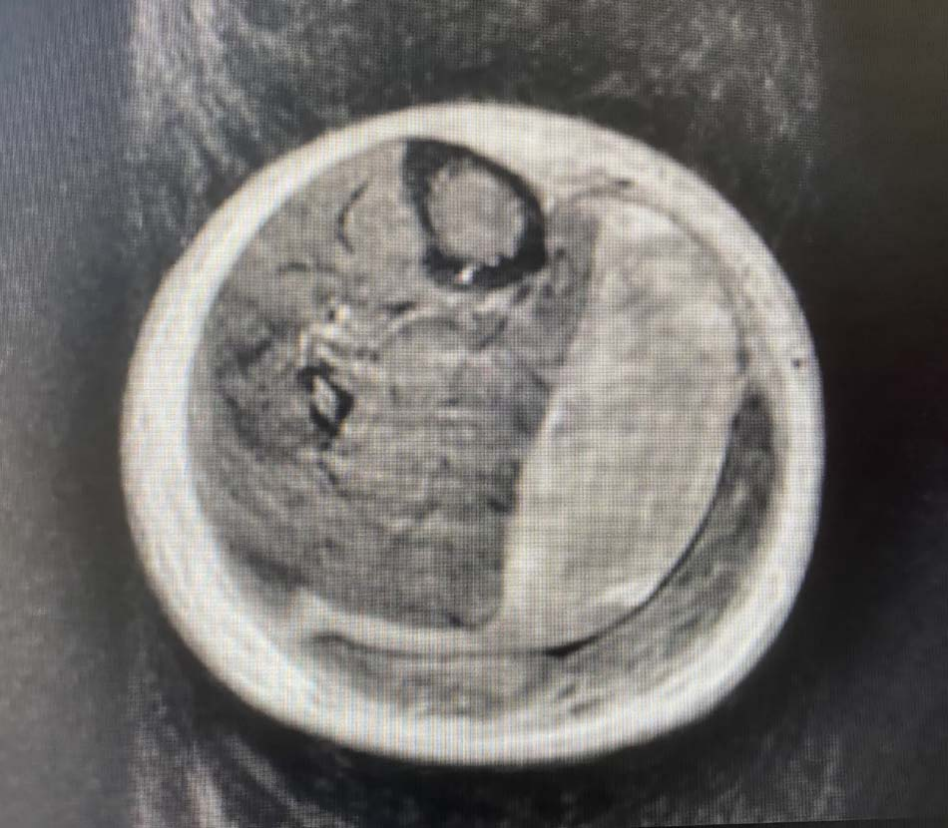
MRI of the RLE showing hematoma in the superficial posterior compartment.
